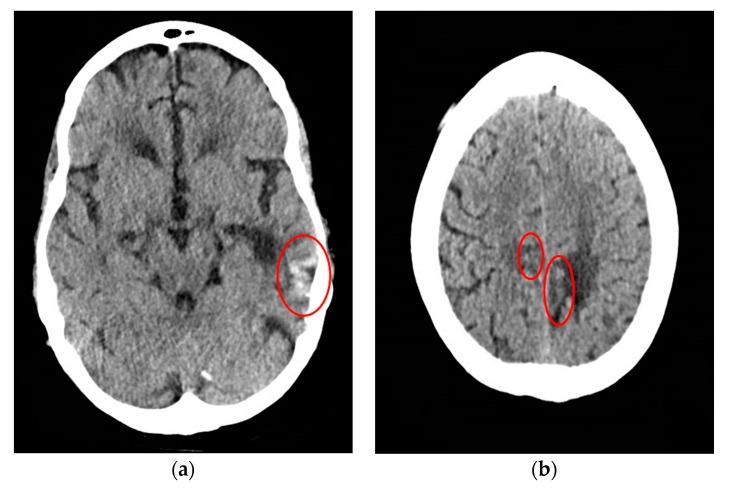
Computed tomography (CT) head of a 61-year-old male with SCNS-DLBCL presenting with acute encephalitis. (a) CT Head shows a small area of inferolateral left temporal density, which correlates with the blood products that were seen in patient’s previous magnetic resonance imaging (MRI) associated with the temporal lobe lesion. There is associated white matter low density, which is similar to the non-enhancing signal on previous MRI; (b) CT Head shows a small area of medial left parietal occipital low density corresponds to the lesion and old blood seen on the MRI. It also shows a very small area of medial right parietal occipital low density corresponds to signal seen on the MRI.
